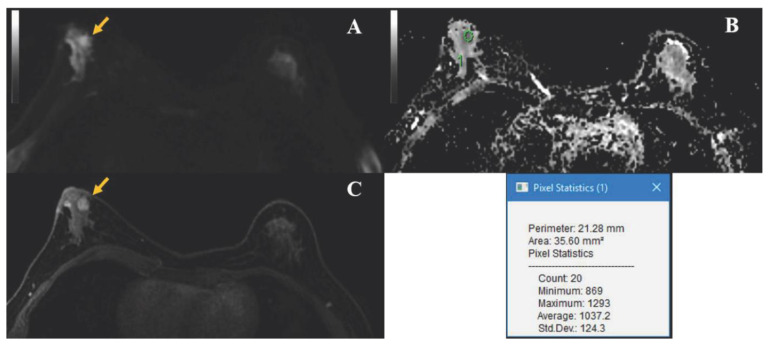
Axial MR images of a 40-year-old woman with a 9 mm benign mass, of which the biopsy yielded intraductal papilloma (yellow arrows). (A) Axial diffusion-weighted imaging (DWI) at a b value of 800 s/mm^2^ shows a hyperintense lesion in the anterior third of the right breast. (B) Correlative parametric apparent diffusion coefficient (ADC) map with a region of interest (ROI) placed within the lesion and ROI information. ADC values are expressed in mm^2^/s. (C) Axial Dynamic contrast-enhanced image depicts a slightly irregular, heterogeneous enhancing mass corresponding to the hyperintense lesion on DWI. This lesion had suspicious characteristics on dynamic contrast-enhanced images and low ADC values and was therefore categorized as BI-RADS 4 in a clinical read, subsequently undergoing a biopsy. Multiparametric radiomics accurately classified the lesion as benign.
